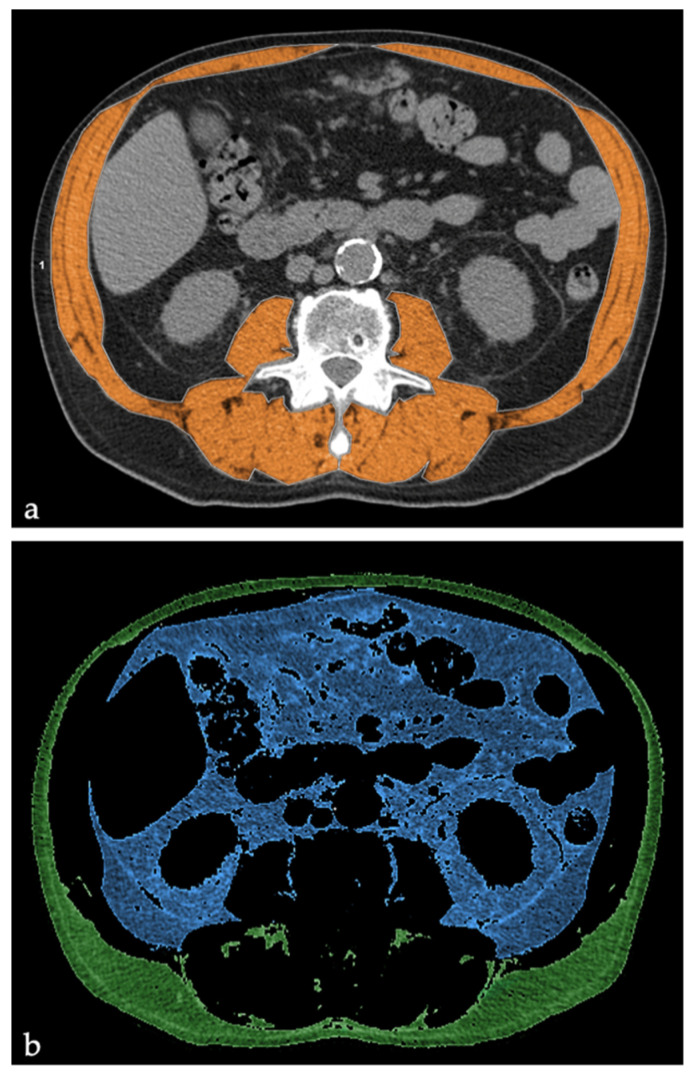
Measurement of body composition parameters with cross-sectional computed tomography (CT) images at the level of third lumbar vertebra. (a) Skeletal muscle area (b) Subcutaneous and Visceral fat areas.
